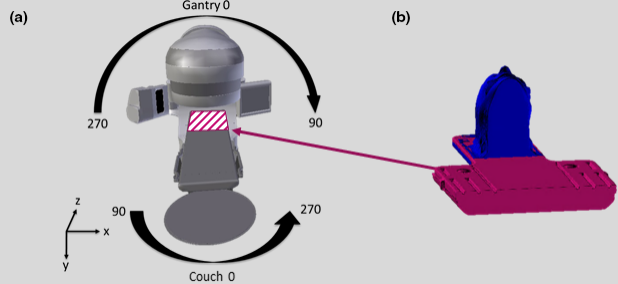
(a) Varian International Electrotechnical Commission gantry and couch coordinate system used, shown with the fixed digital imaging and communications in medicine patient coordinate system. (b) Computed tomography body contour containing the head of the Orfit board with the registered additional couch structure.
